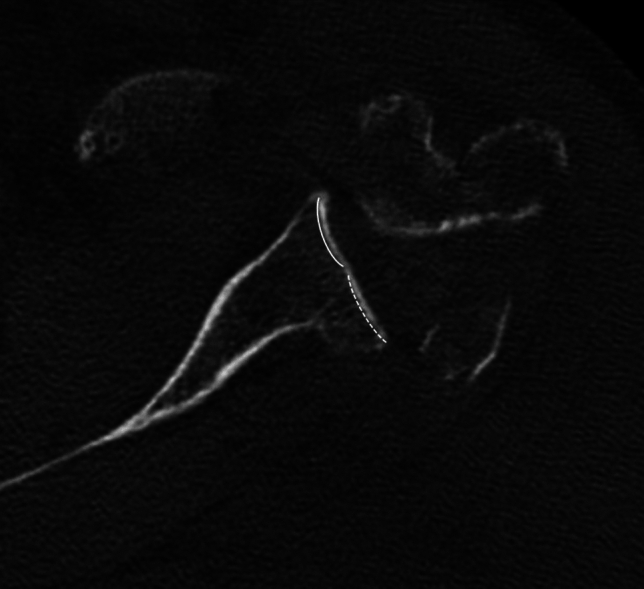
Axial CT image through the mid glenoid showing Walch class B2 morphology with a biconcave articular surface, including the expected concavity anteriorly (solid line) and secondary concavity posteriorly from osseous wear (dashed line)
